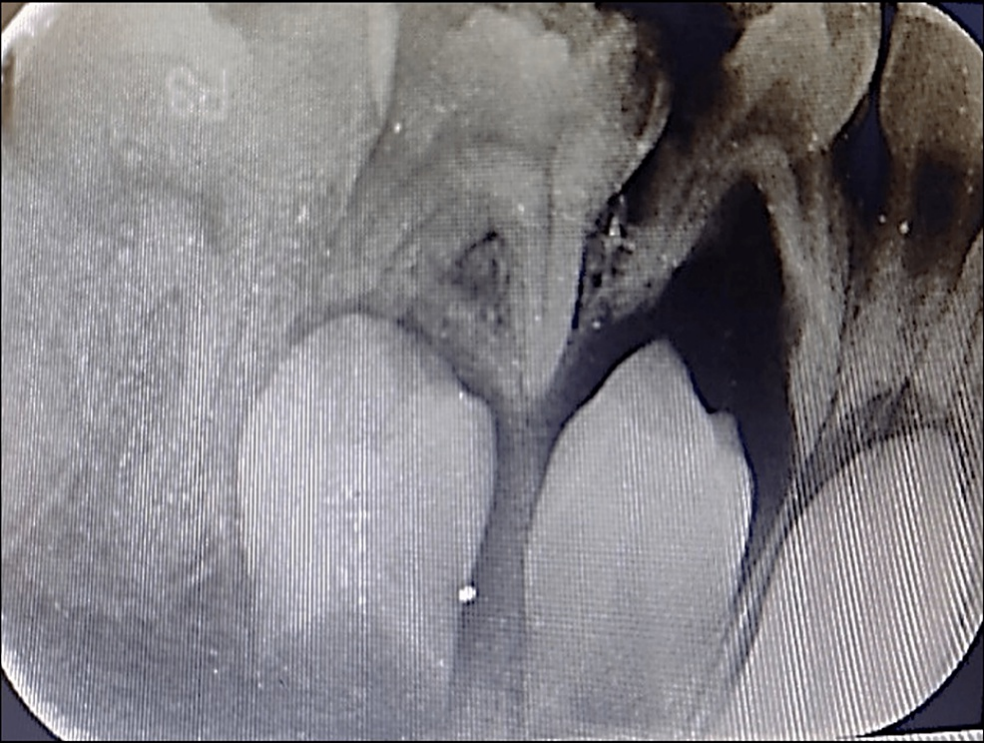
The IOPA radiograph revealing a periapical radiolucent lesion extending from the distal root of 83 to the mesial root of 85. IOPA, intraoral periapical
